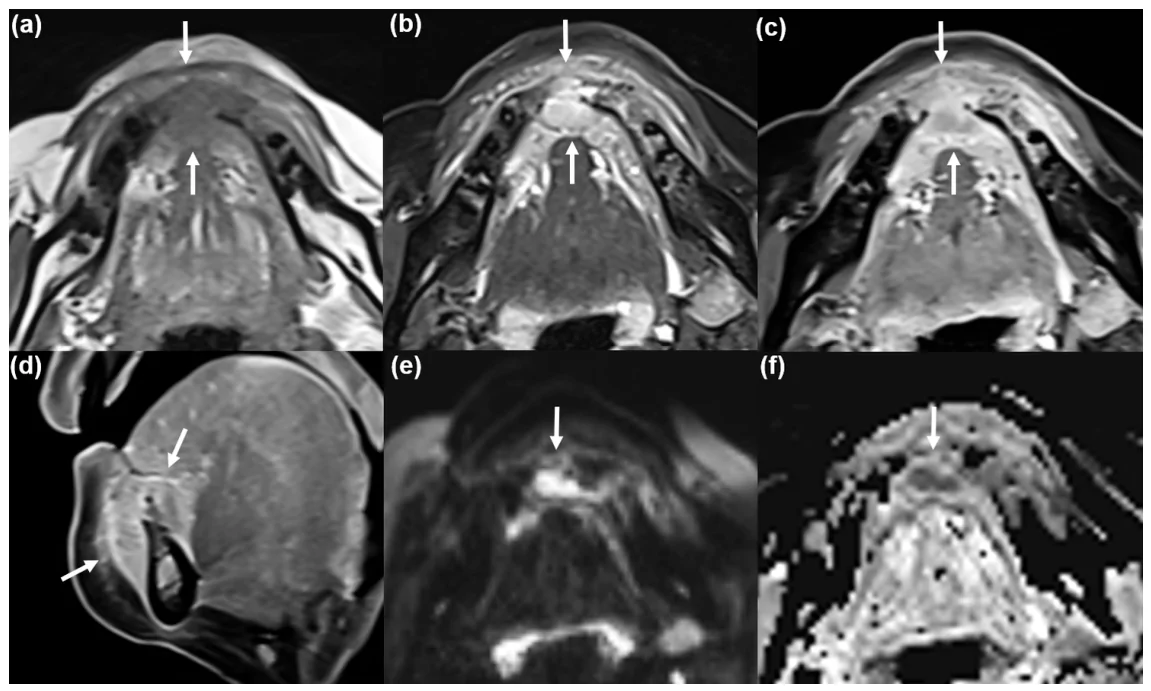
MRI findings. (a) Axial T1-weighted image shows a hypointense soft-tissue gingival mass with bone involvement at the mandibular symphysis. (b) Axial fat-suppressed T2-weighted image shows inhomogeneous high signal intensity of the lesion with focal tumor extension to the anterior floor of the mouth (in particular, sublingual glands). (c,d) Axial (c) and sagittal (d) fat-suppressed contrast-enhanced T1-weighted images show heterogeneous enhancement of the tumor with abutment of the adjacent floor of mouth musculature. (e,f) Axial diffusion-weighted image (DWI) (e) and the corresponding apparent diffusion coefficient (ADC) map (f) demonstrate diffusion restriction within the lesion, with a mean ADC value of 0.87 ± 0.23 × 10^−3^ mm^2^/s. Arrows in the images indicate the lesion.
